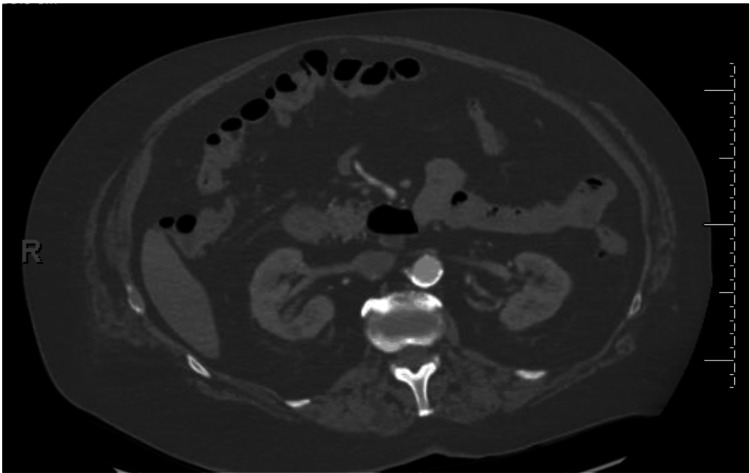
CT abdomen The kidneys and spleen are within normal limits, with no free fluid, free air, or evidence of bowel obstruction.
